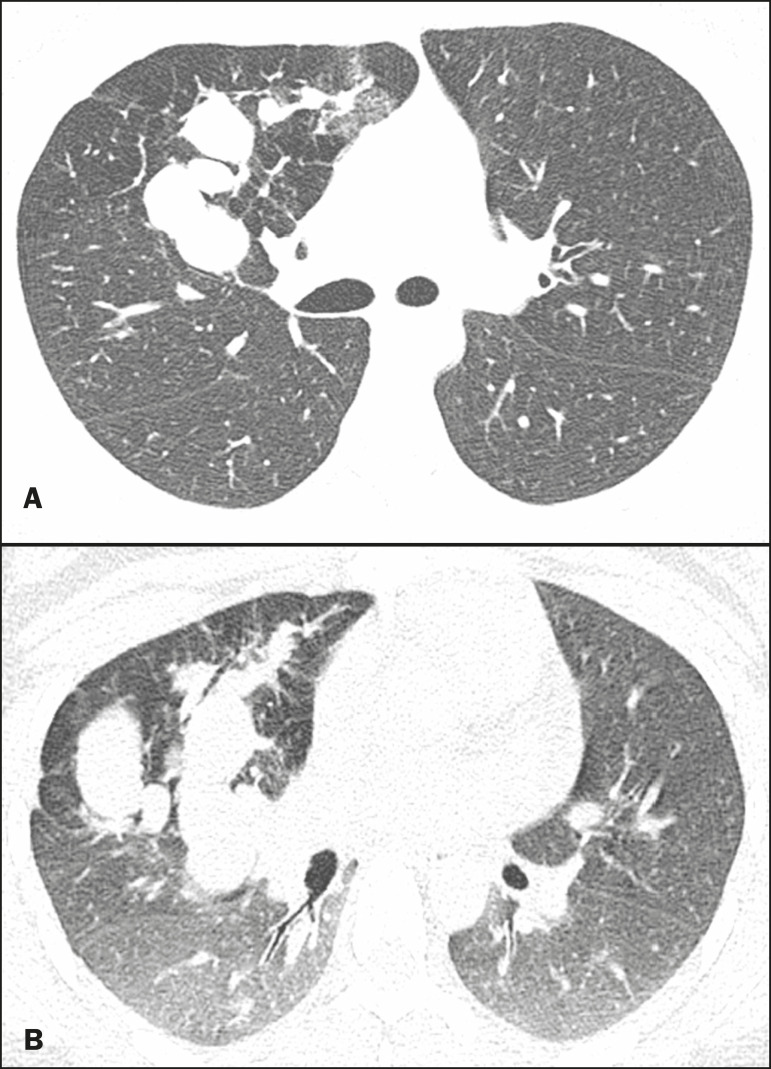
Male, 27-year-old, presenting with cough symptoms. Axial chest CT scan (A), showing branching opacities in the right upper lobe, with adjacent lung hyperinflation, best visualized in an expiratory sequence (B).
